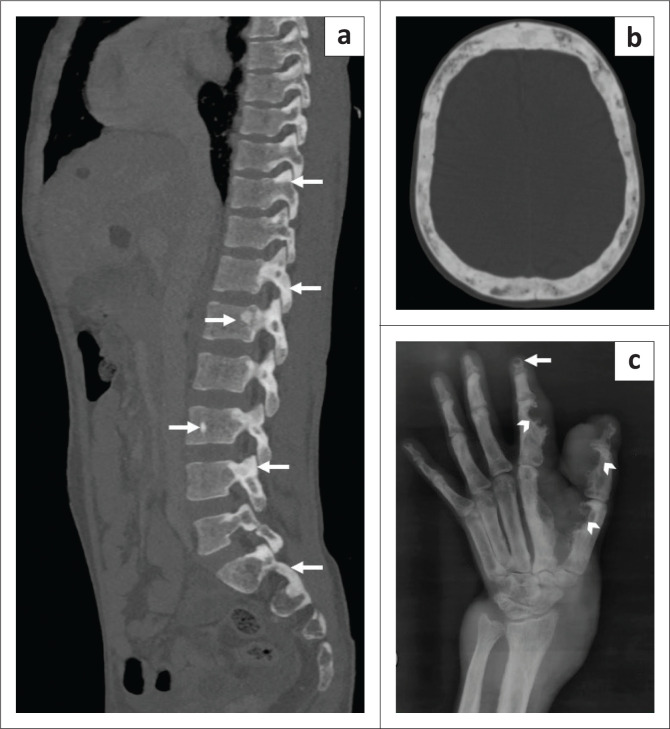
Bone manifestations of tuberous sclerosis complex. Reformatted sagittal computed tomography (CT) in bone window (a) shows patchy and round sclerotic lesions (white arrows) involving the posterior elements and vertebral bodies at multiple levels in the thoracolumbar spine. The axial bone window of the CT head (b) demonstrates a diffusely thickened calvarium with patchy sclerosis. An oblique radiograph of the left hand (c) shows typical intra-osseous cysts in the terminal phalanges (white arrows). The left thumb and second digit (b) additionally show multiple cortical defects or scalloping (arrow heads) and periostitis with associated soft-tissue components, likely representing large cutaneous angiofibromas or hamartomas.
